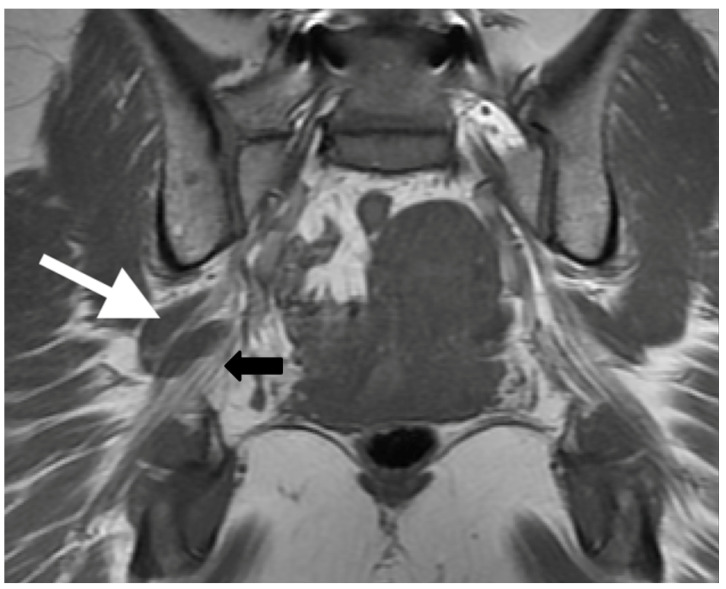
Coronal T1-weighted sequence of the bilateral sciatic nerves showing a type II variant with a common peroneal nerve component piercing a bifid piriformis (white arrow), tibial nerve component running in normal position anterior and inferior to piriformis (black arrow).
